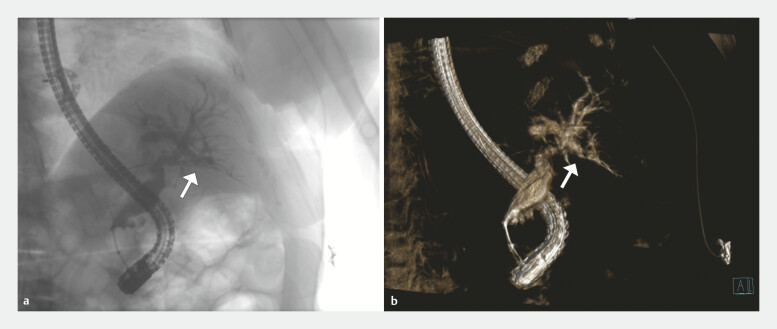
CBCT imaging of the biliary system with white arrows indicating the target bile duct.
